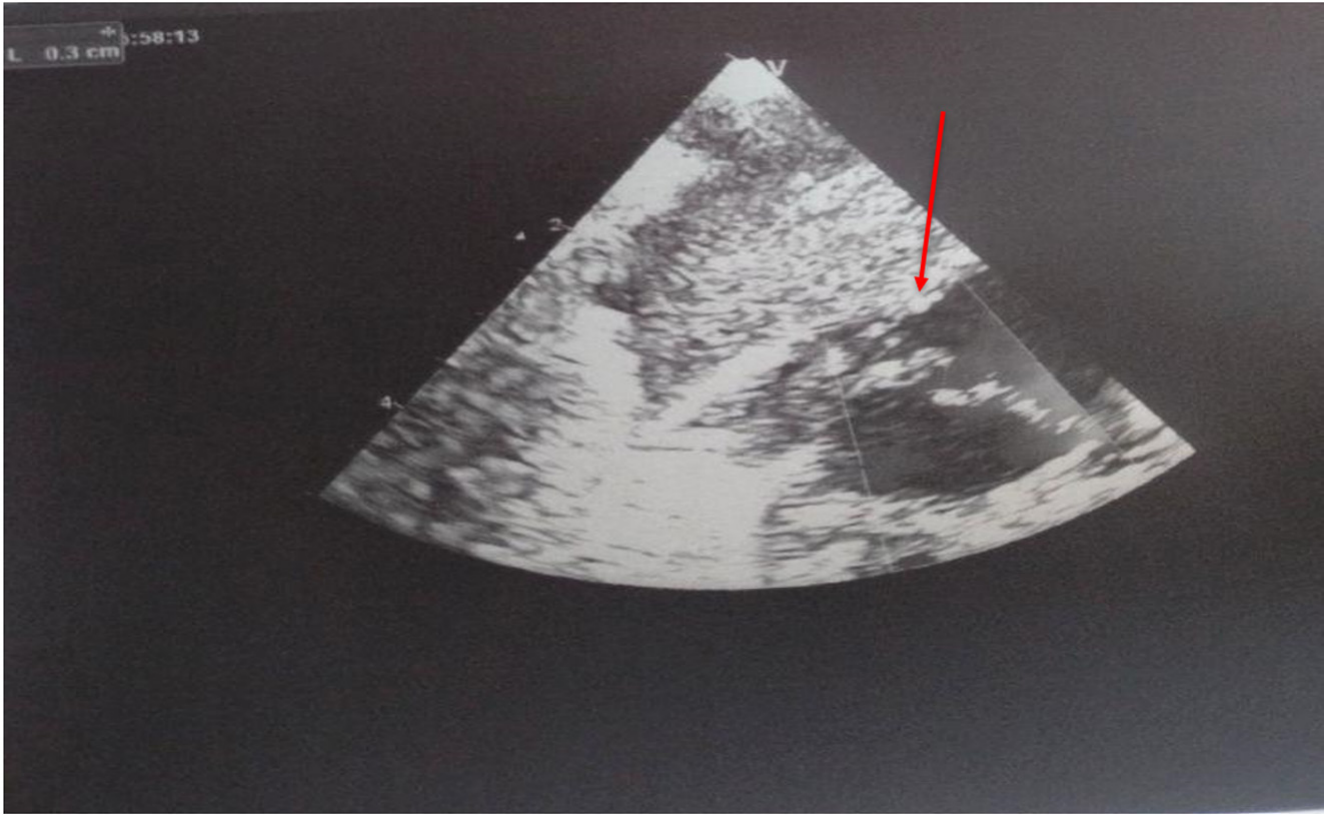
Echocardiography shows patent PFO. Levocardia, Situs solitus, normal intracardiac anatomy, patent foramen ovale (PFO) 3 mm with a left to right shunting, qualitatively normal right ventricular size, and systolic function, Normal left ventricular size and systolic function, and no evidence of significant pericardial effusion.
